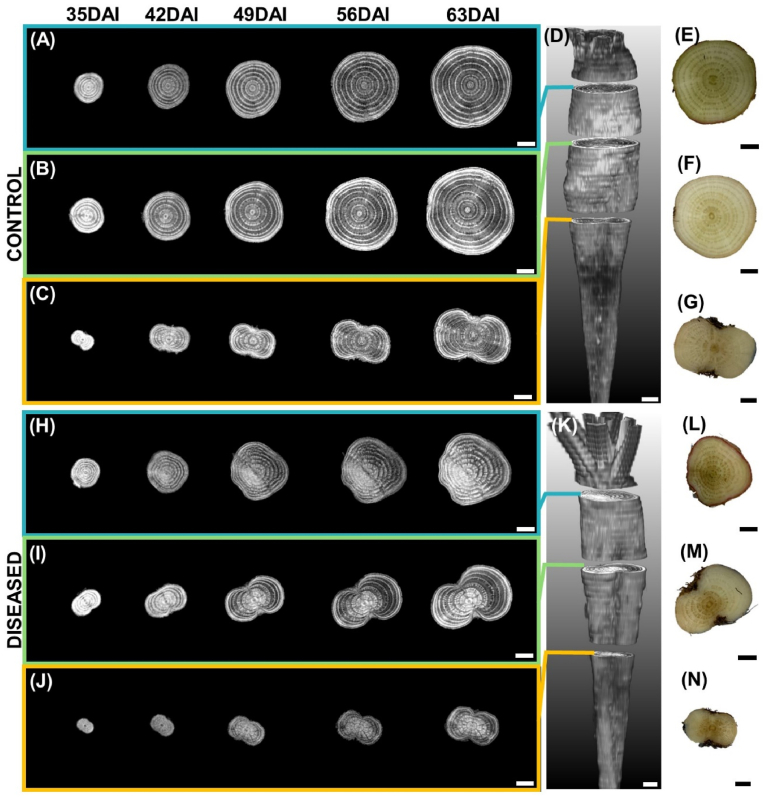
Examples of cross-sectional anatomical features at three vertical positions of control taproot C1 (A–G) and diseased taproot D1 (H–N). Grey images (A, B, C, H, I, J) represent slices of MRI acquired at five time-points after inoculation (35, 42, 49, 56 and 63 DAI). (D, K) indicate vertical positions where slices were obtained for control and diseased taproot, respectively. (E, F, G, L, M, N) RGB images show a cross-section of the same control (E, F, G) and diseased (L, M, N) taproots after destructive harvest at 63 DAI. Brownish discoloration in (L, M, N) shows SBR symptoms. Bars, 0.5 ​cm.
